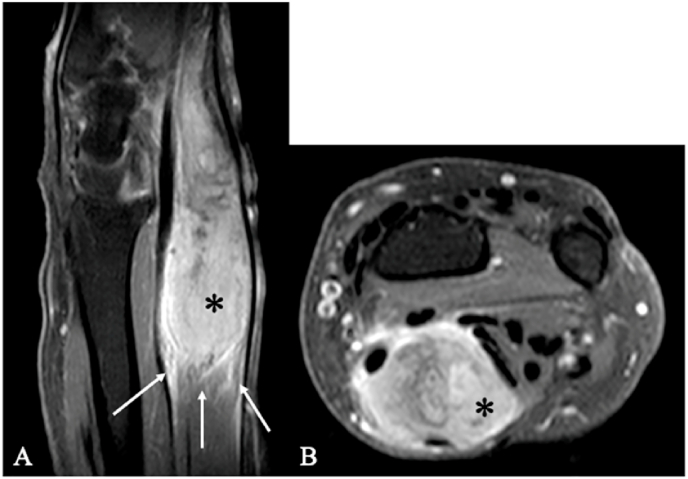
Plexiform xanthoma of the right wrist. (A) Longitudinal contrast-enhancing MRI of a 59-year-old woman shows a multilobulated lesion along the volar wrist with infiltration of the surrounding soft tissues (B). Axial image shows the lesion occupying the carpal tunnel (asterisk). A schwannoma of the median nerve was initially suspected on imaging. Intraoperatively, the tumor infiltrated the median nerve with contiguous spread into adjacent muscle. A complete resection and reconstruction were performed using a sural nerve interposition graft, resulting in postoperative new paresis and sensory deficits.
